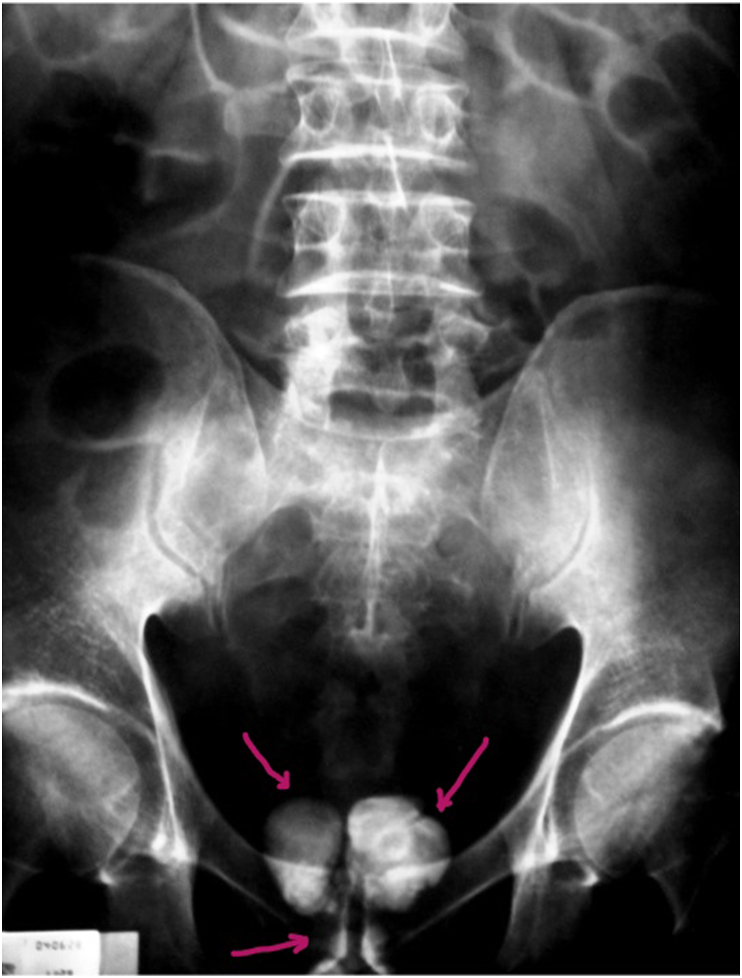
KUB radiography: Bilobed opacity of calcic tone projecting on the prostatic cavity, two other opacities projecting on the path of the urethra.
